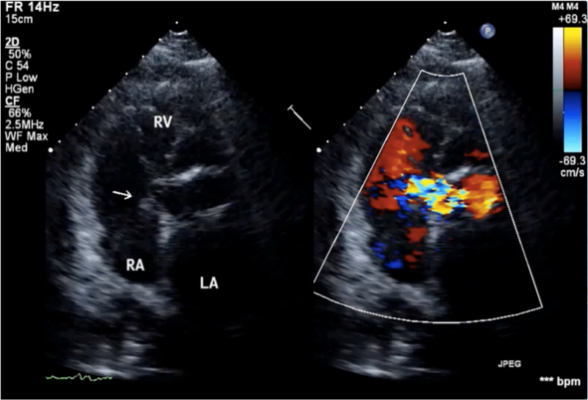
Transthoracic echocardiography (TTE) at the parasternal aortic short axis view. *Left* two-dimensional TTE, demonstrating the vegetation attached to the annulus of the aortic valve (*arrow*). *Right* color Doppler TTE, demonstrating the abnormal flow into the right atrium
